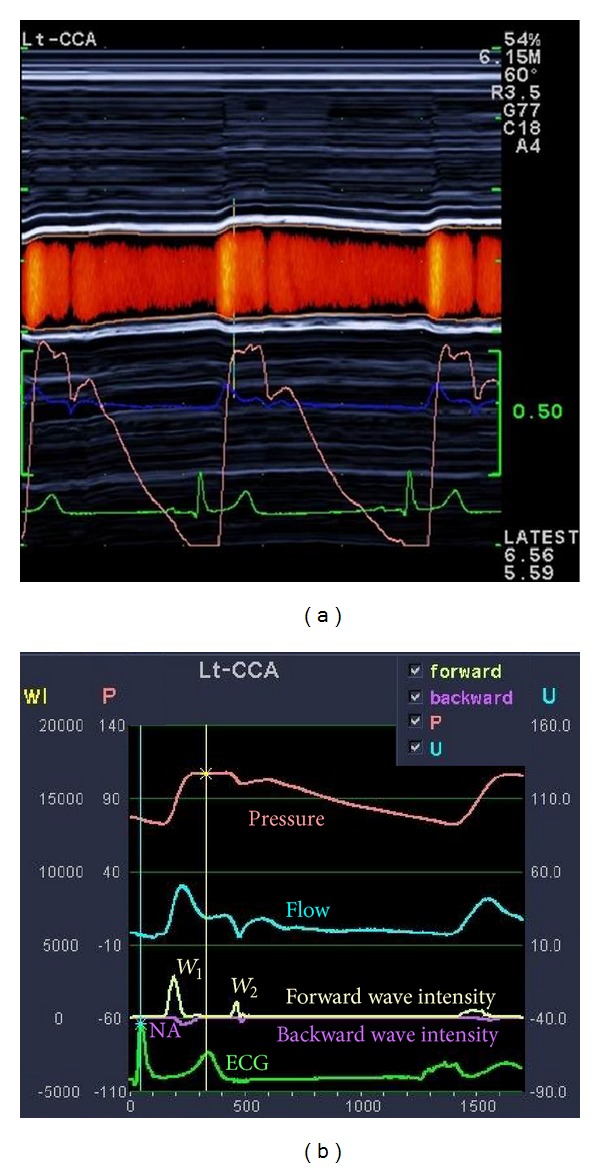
(a) Simultaneous measurement of CCA diameter changes (pink lines) creating the distension waveforms (analogous to a pressure waveform) and CCA blood flow velocity waveforms (dark blue) from echo tracking and color Doppler. (b) Representative view of results obtained from WIA. From top to bottom: distension (pressure) waveform, flow velocity waveform, wave intensity analysis (*W*
